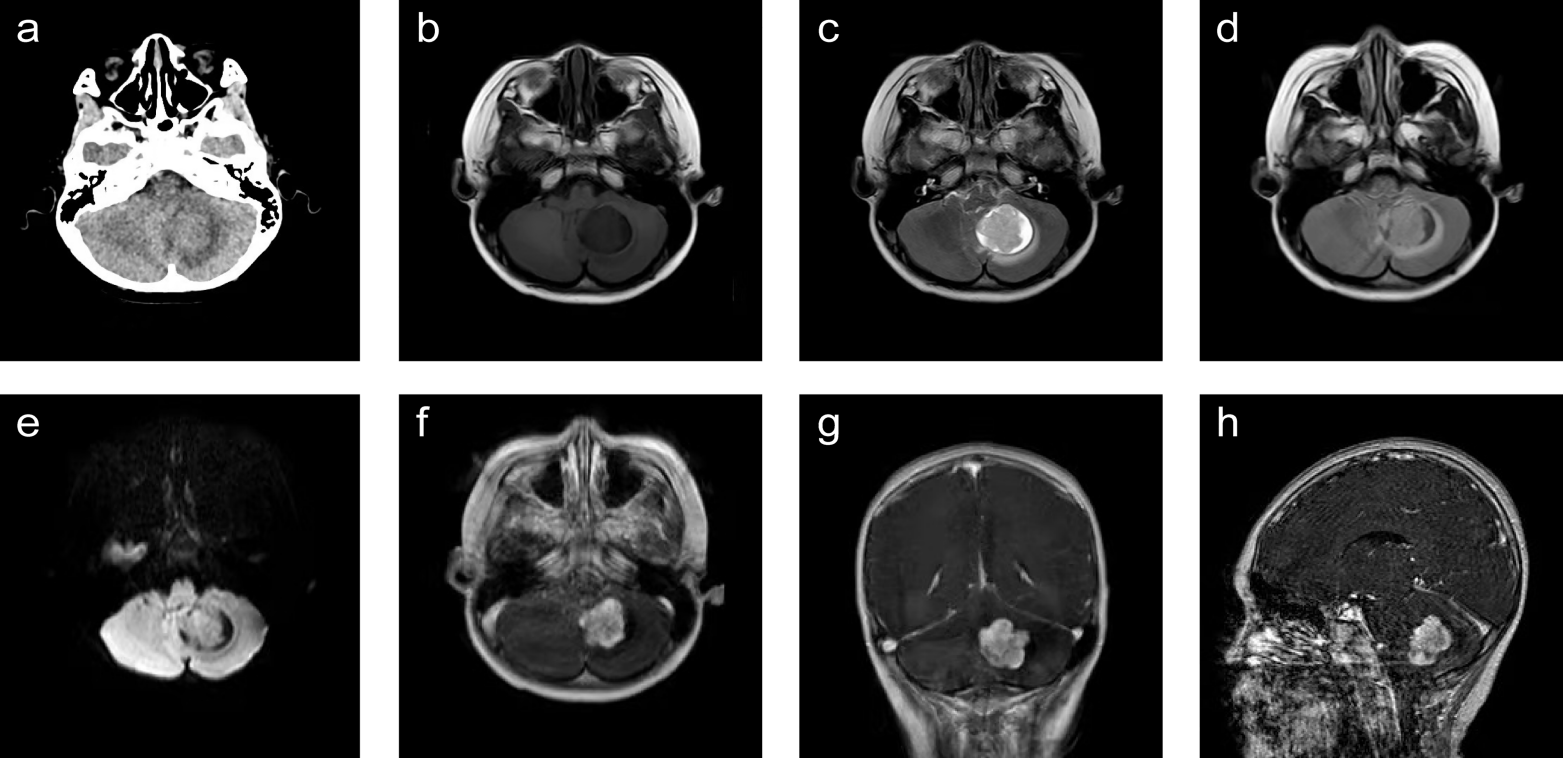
Radiological evaluation of yolk sac tumor before operation. A noncontrast CT scan showed a slightly hyperdense mass located in the left cerebellar hemisphere (A). The tumor was slightly hypointense on axial T1-WI (B) and slightly hyperintense on axial T2-WI, FLAIR and DWI (D, E). Postcontrast (gadolinium-enhanced) axial, coronal and sagittal MRI showed prominent homogeneous enhancement (F‒H). The fourth ventricle was compressed and narrowed. Mild edema was noted around the lesion. (B–H). WI, weighted imaging.
